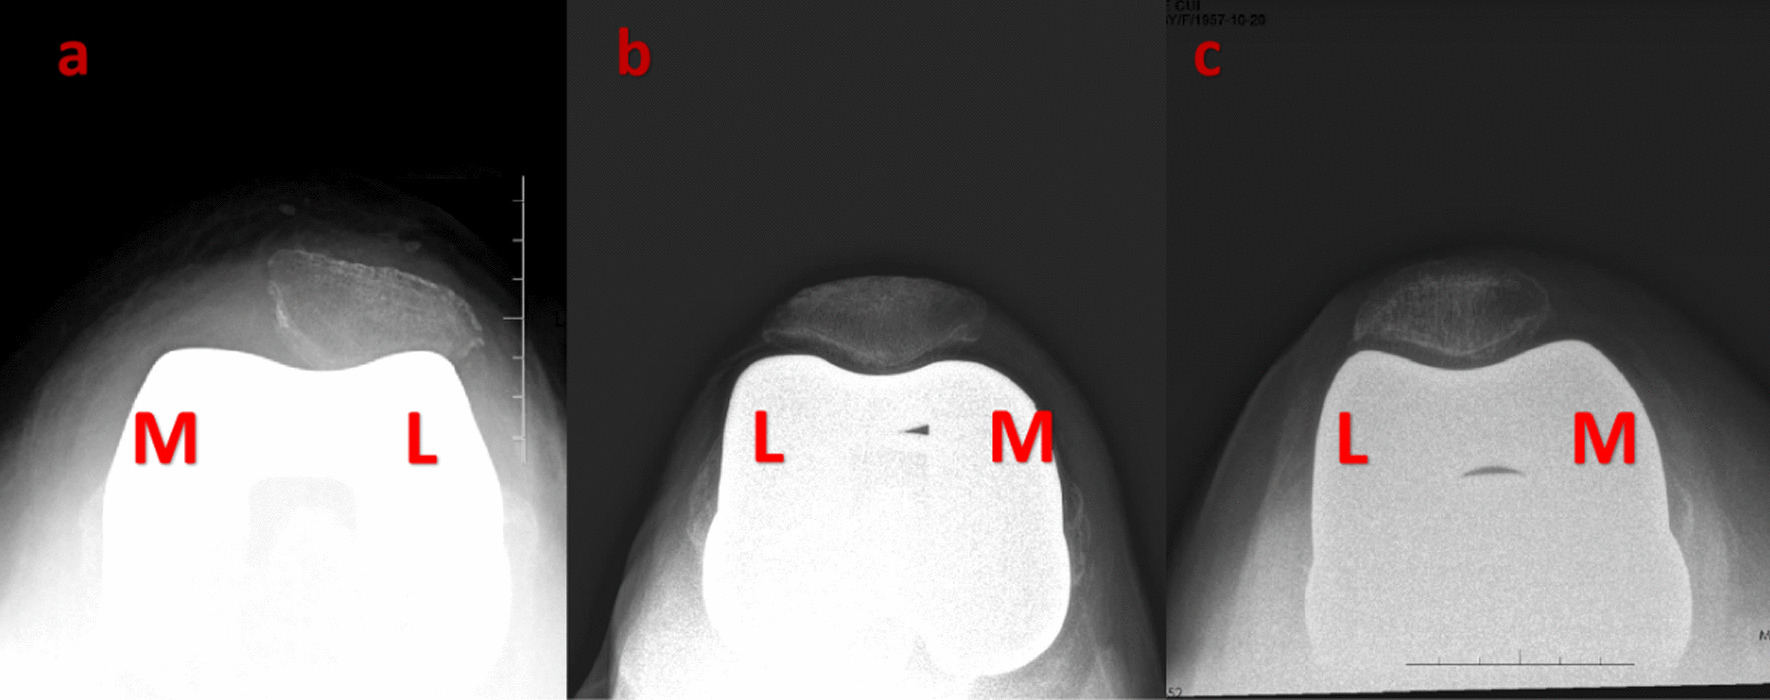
Postoperative follow-up axial patellar X-rays. a. Genesis II b. Triathlon c. Gemini MK II. Abbreviations: M, medial; L lateral
